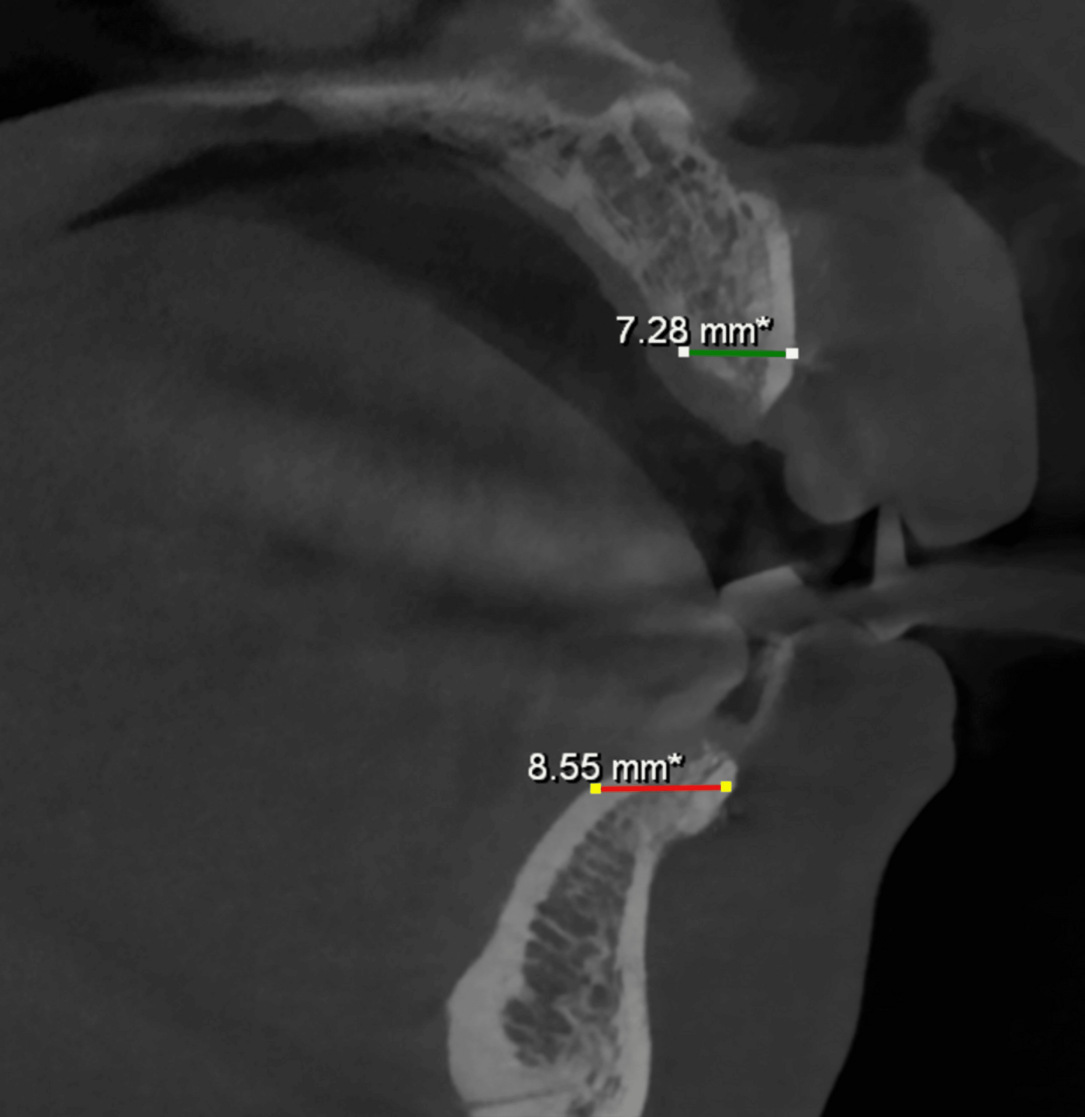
Postoperative CBCT (six months later) showing sagittal views of the maxillary and mandibular ridge widths, measuring 7.28 and 8.55 mm, respectively. CBCT, cone-beam computed tomography
